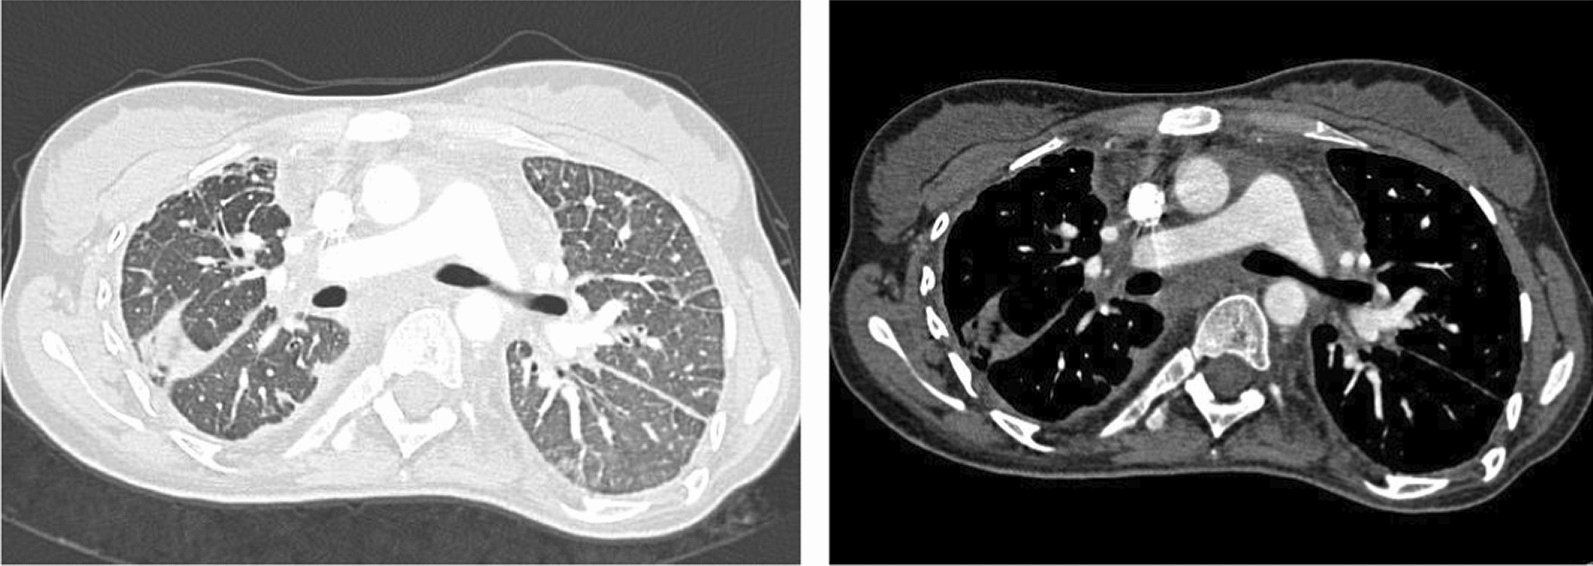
CT-Thorax: mediastinal mass, pleural thickening and septal thickening in PL
